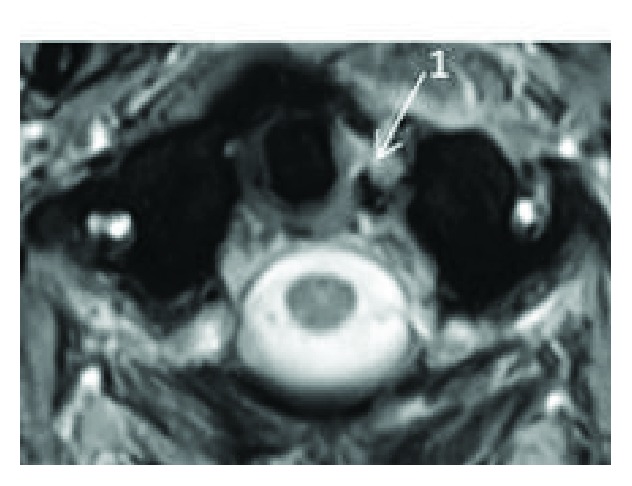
Cervical MRI axial view showing complete disruption of the left transverse ligament (1).
